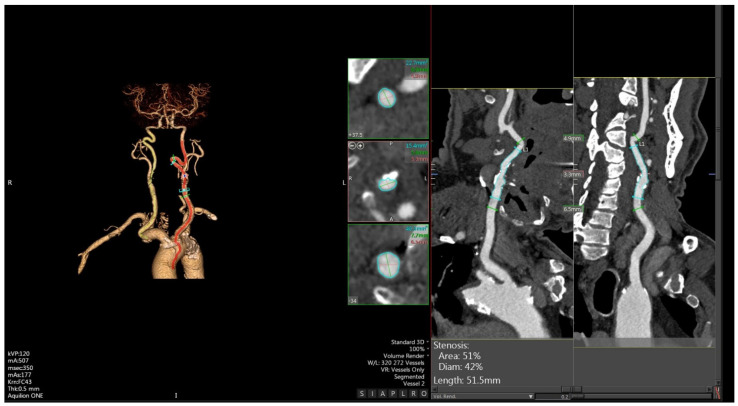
Shown are 2D and 3D reconstructions of a nonsignificant calcific plaque by CTA.
